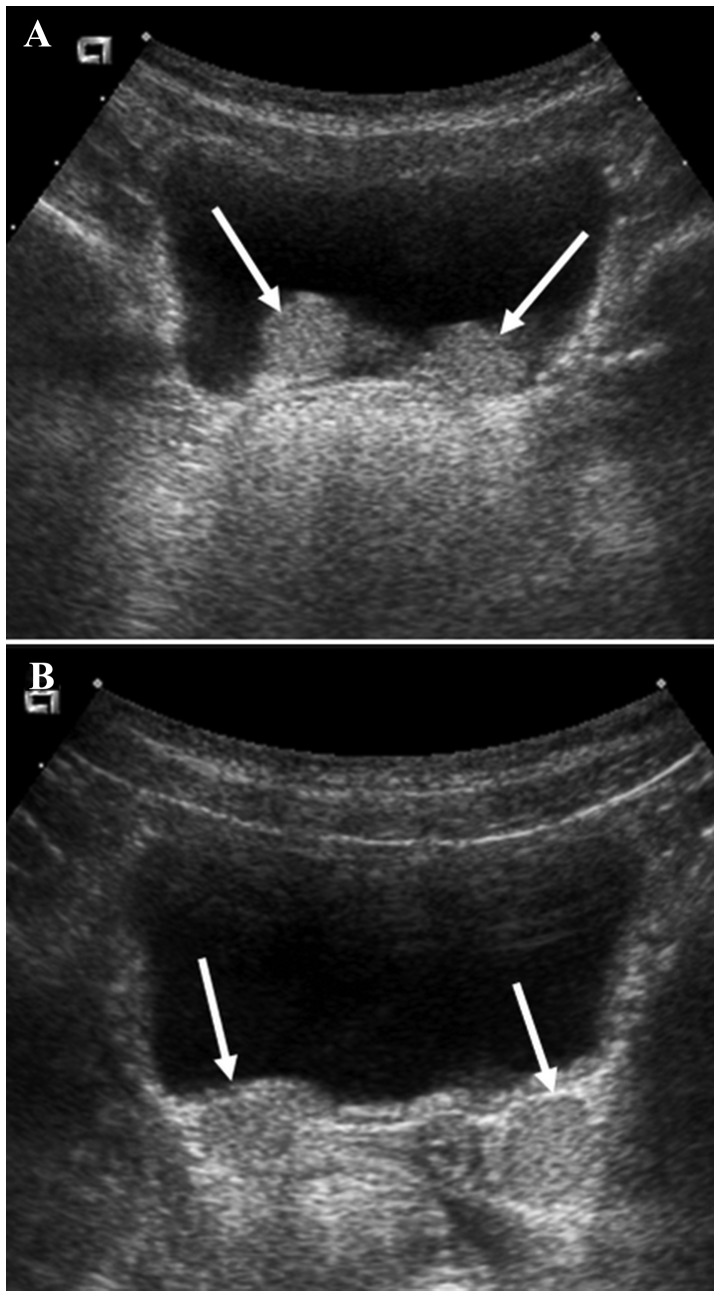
Ultrasonic images of an injection nodule. A nodule is defined as (A) a protruding mass lesion or (B) an echogenic mass, as indicated by the arrows.
